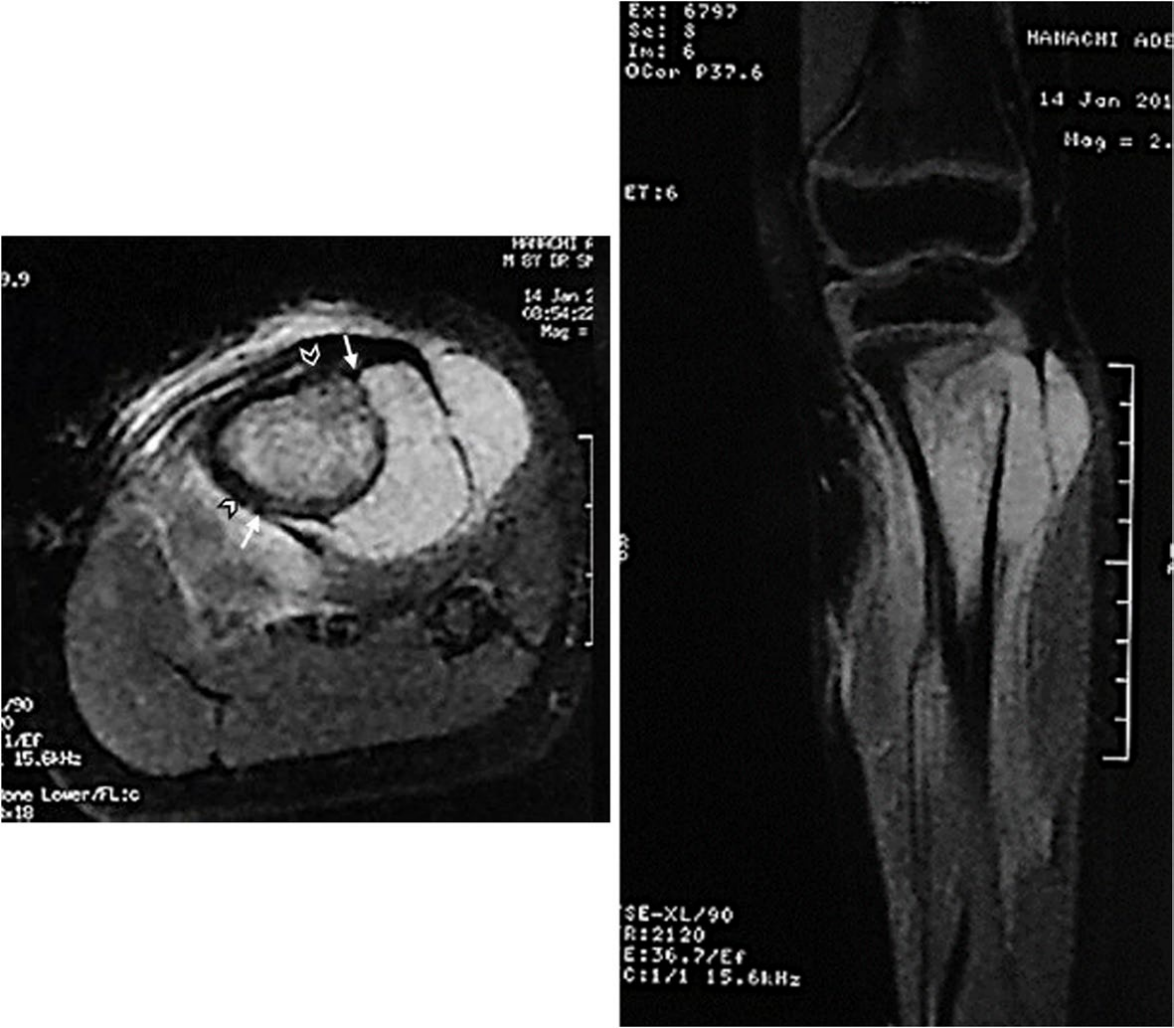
Ewing sarcoma of the proximal tibia in an 8-year-old boy. MR images show an extra-compartmental involvement and a partially circumferential (medial) respect for the CPU. Footnotes: arrows: axial edges; chevrons: level of the periosteal section
